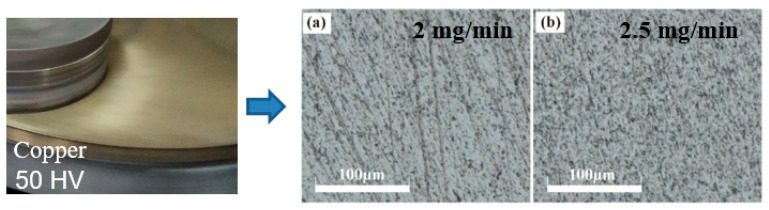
Optical images after lapping using copper disc: (a) common lapping; (b) ultrasonic-assisted lapping.
